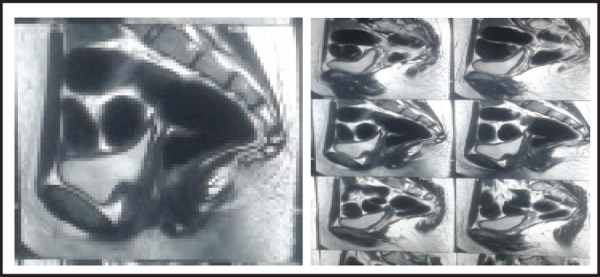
Magnetic resonance imaging examination sagital plane cut showing absence of uterus and ovaries.
